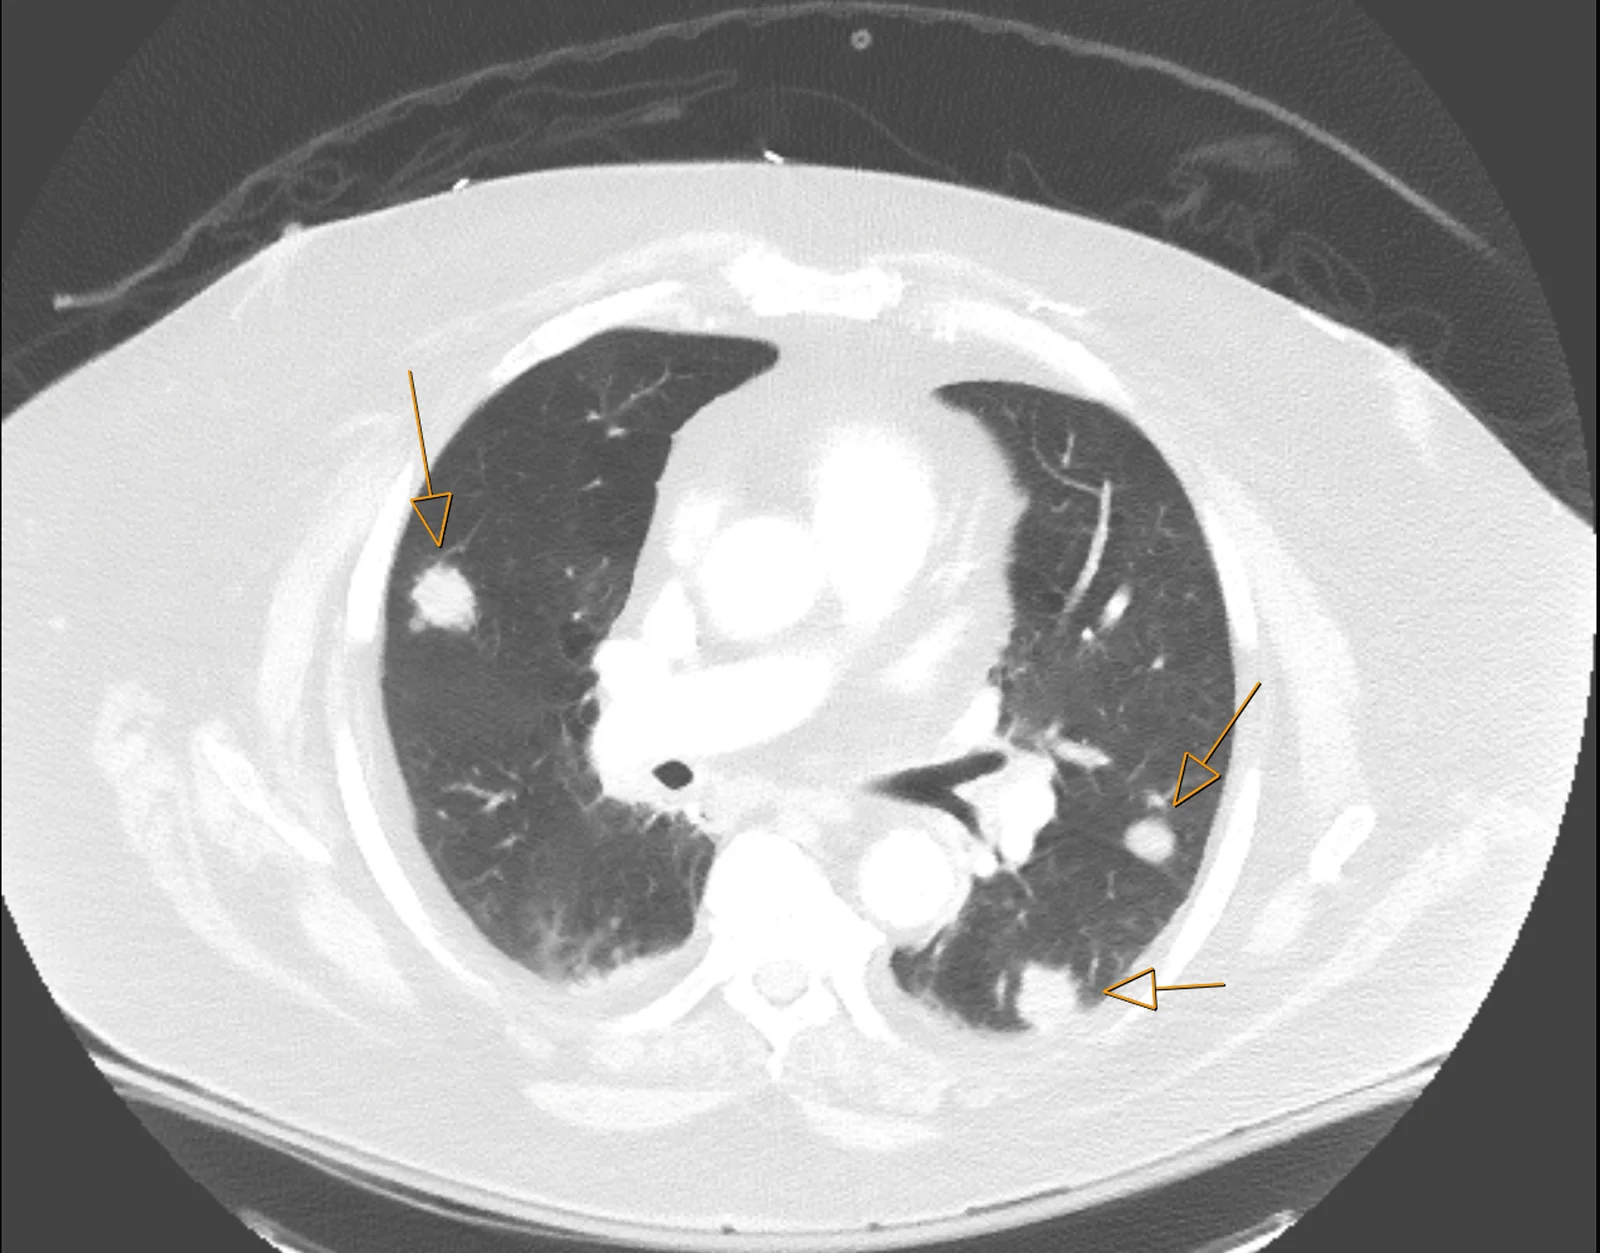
Computed tomography of the chest demonstrating multiple lung nodules (arrows).
